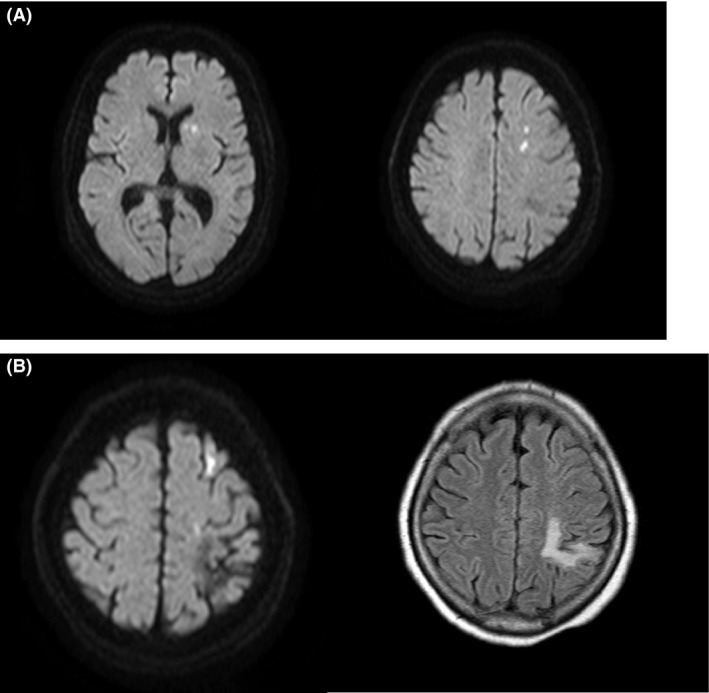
Magnetic resonance imaging sequences of the brain. Fresh multiple infarctions were observed in the cerebral white matter and basal ganglion as restricted diffusion (A), which were accompanied by an old infarction in the left parietal lobe (B).
